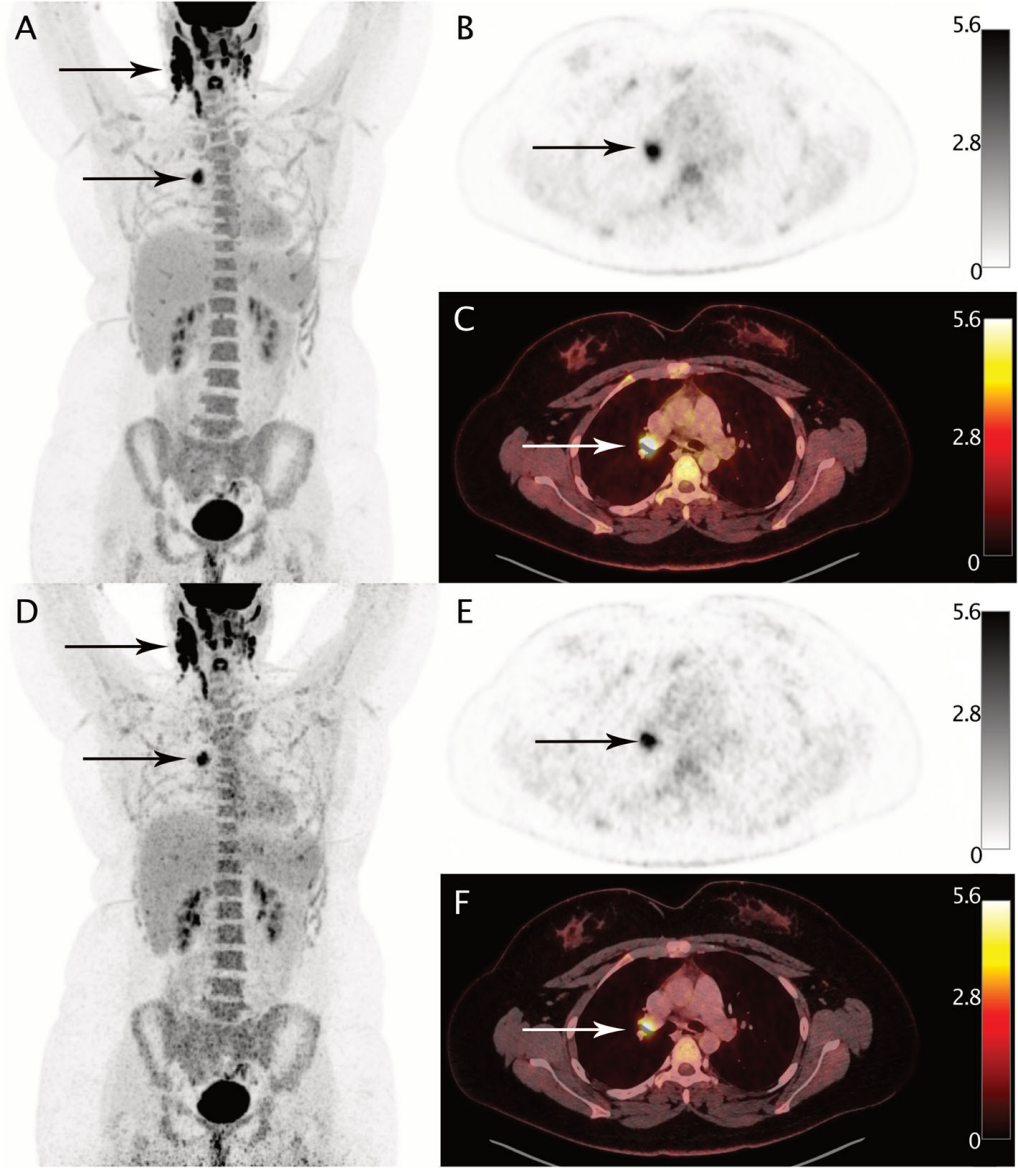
A 25-year-old patient with biopsy-proven lymphoma undergoing ^18^F-FDG-PET/CT before treatment. Panels a, b, and c show images acquired with the reference acquisition protocol, panels c, d, and e show image acquired with reduced emission time, all reconstructed with OSEM-PSF+TOF (4i). Axial slices (b, c, c, and f) and maximum intensity projections (a and d) reveal vital lymphoma manifestations in cervical and mediastinal lymph nodes. All lesions visible with standard acquisition protocol were also detectable after a threefold reduction of scan time duration. Values left to the color bares are in SUV units
